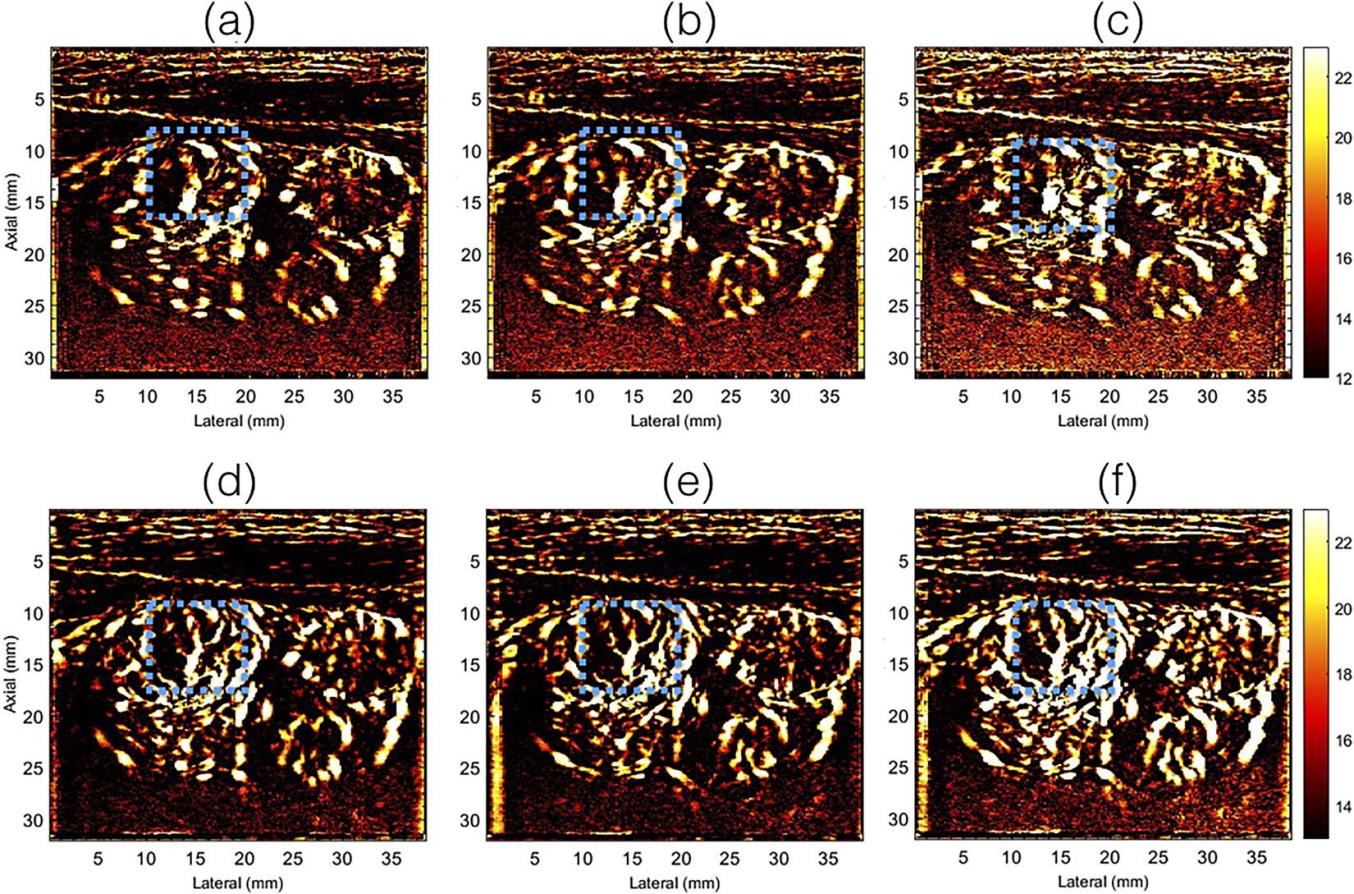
Displays the half- and full-ensemble PD images obtained using clutter filtered Doppler ensemble, without (a–c) and with (d–f) motion correction. The half ensemble PD images are displayed in (a,b,d,e), and the corresponding full-ensemble PD images were displayed in (c,f). The blue ROI indicates a region for closer observation and comparison.
